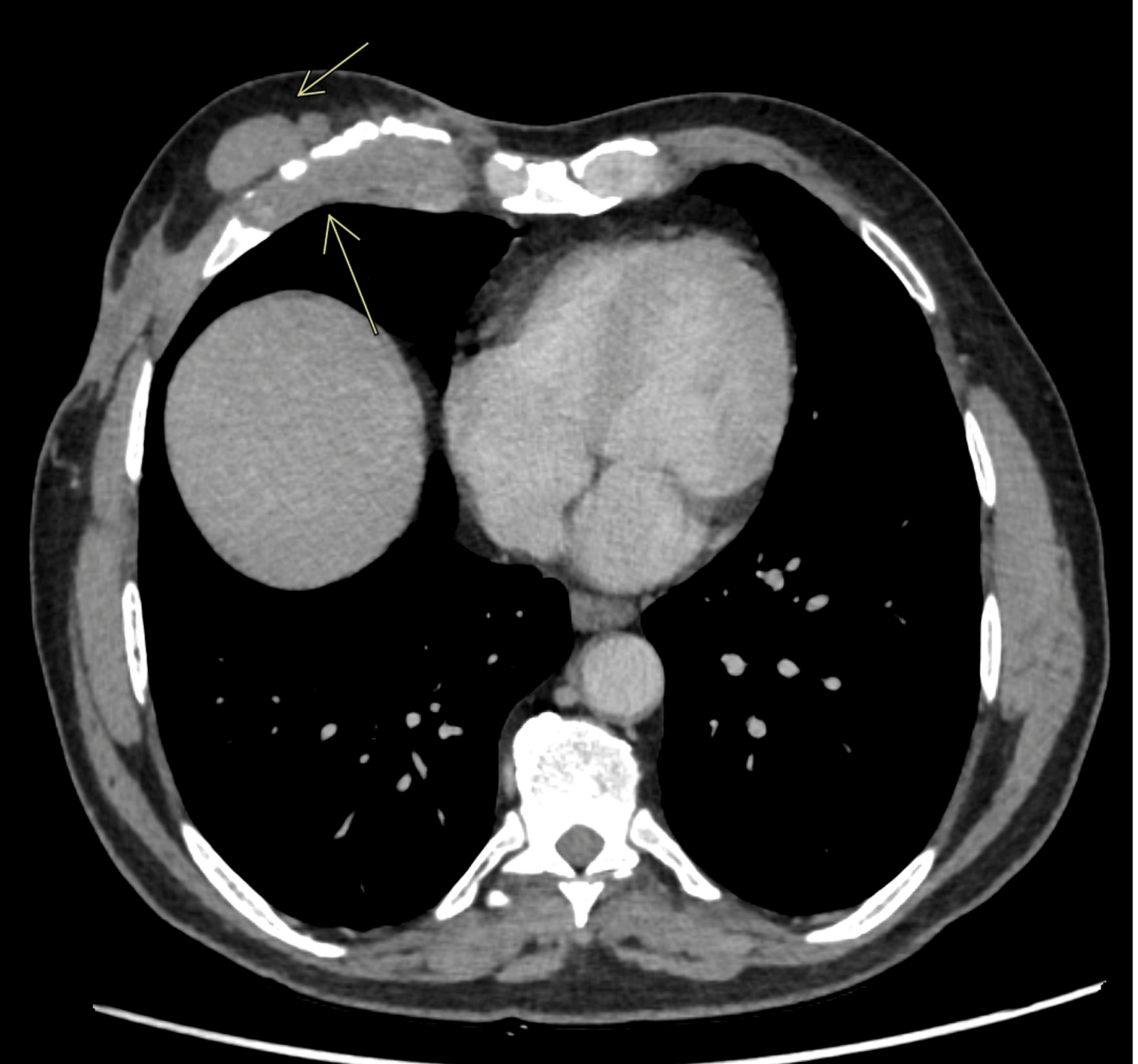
Contrast-enhanced computed tomography (CECT) chest findings Demonstrates soft tissue mass around the right fifth costal cartilage and bony erosion with fragmentation of cartilage. Well-defined soft tissue nodules were also noted in the anterior abdominal wall, as shown by the arrow.
